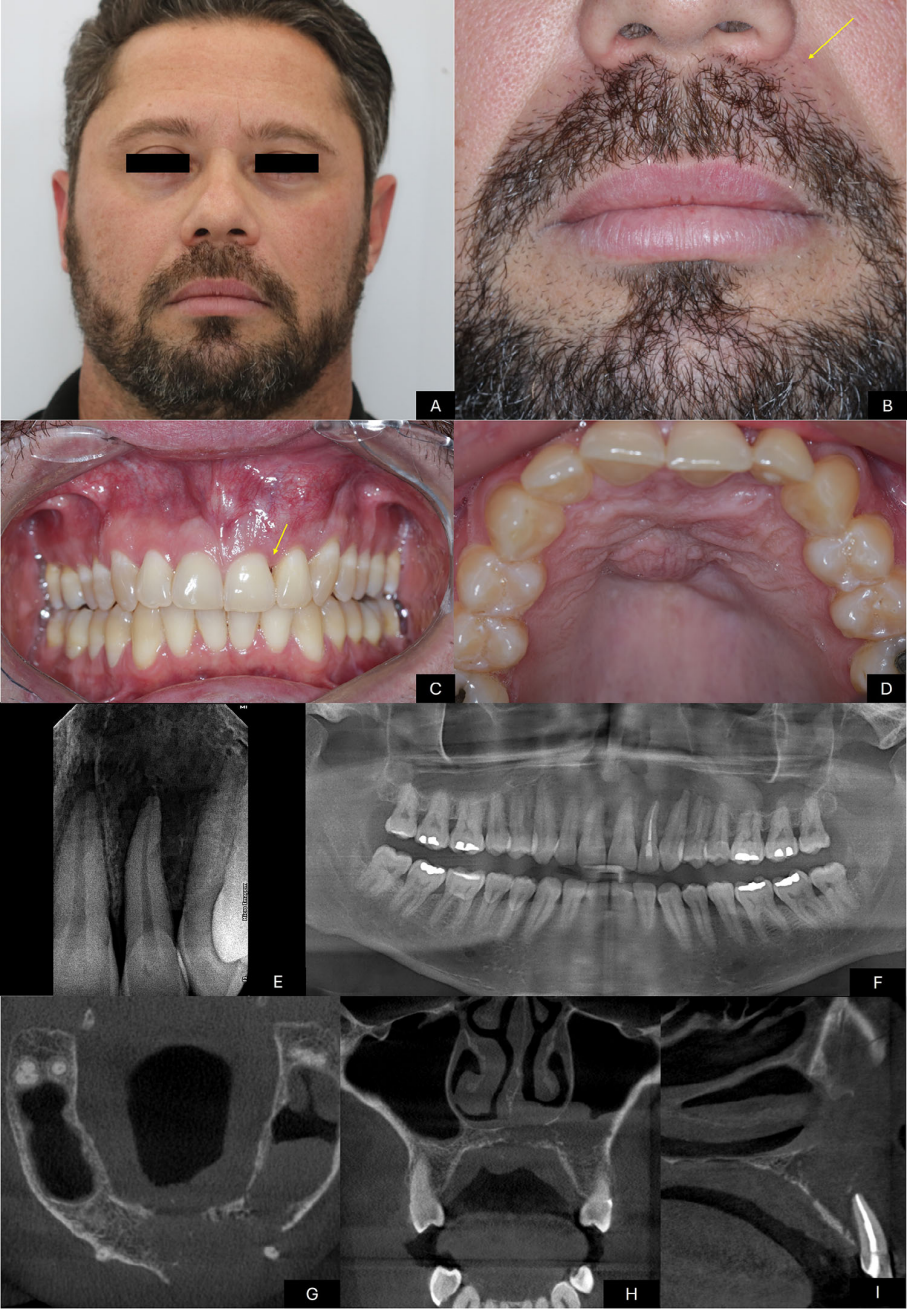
Initial clinical and radiographic aspects. (A‐B) Initial clinical examination revealing mild facial asymmetry due to a localized swelling in the upper left lip region (yellow arrow). (C) Intraoral swelling in the anterior region of the left maxilla, resulting in a reduced maxillary vestibular fornix, with a purplish hue and a telangiectatic surface. A decrease in the interdental papilla is observed in the interproximal region between teeth lateral and central incisors (yellow arrow). (D) Swelling along the midline of the hard palate. (E) Periapical radiograph demonstrating a diffuse radiolucent area at the periapex of tooth #7. (F) Panoramic radiograph showing a poorly defined radiolucent image in the periapical region of teeth. Tooth lateral incisor presents previous endodontic treatment. (G) Axial slice of computed tomography (CT) revealing an expansile hypodense lesion in the anterior maxillary region, associated with cortical bone disruption. (H) Coronal CT reconstruction demonstrating hypodensity in the left maxillary sinus and the inferior portion of the nasal cavity, associated with bone destruction. (I) Sagittal CT reconstruction showing satisfactory endodontic treatment of tooth lateral incisor, associated with bone destruction, cortical disruption, and root resorption.
